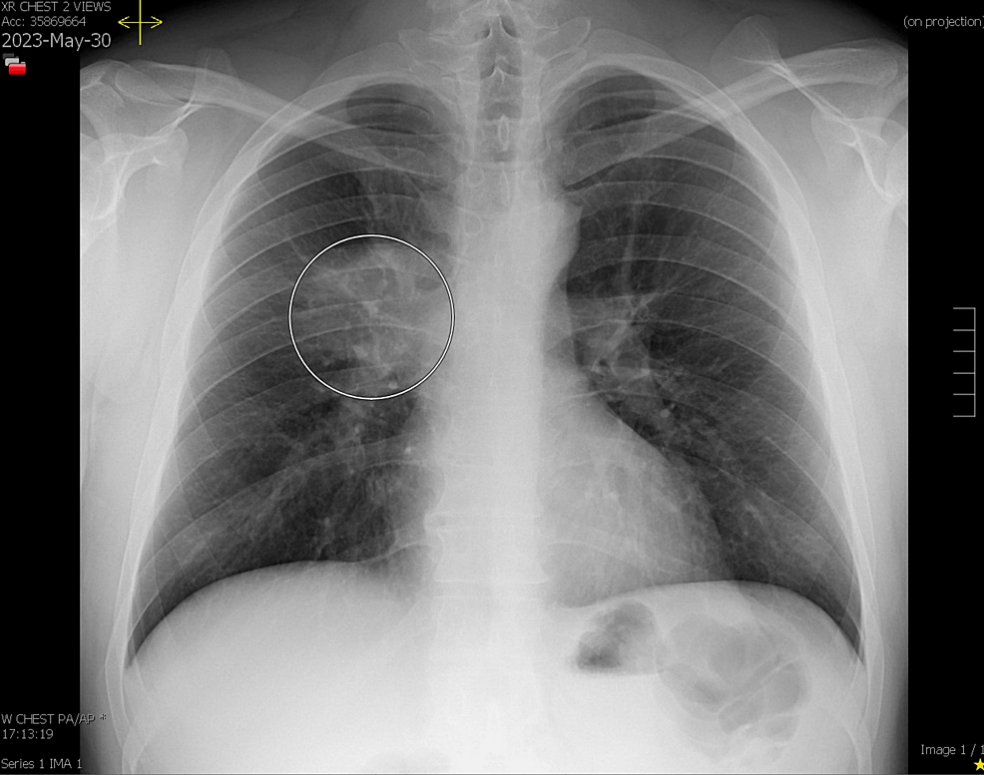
Chest X-ray depicting right upper lobe consolidation (posteroanterior view)
